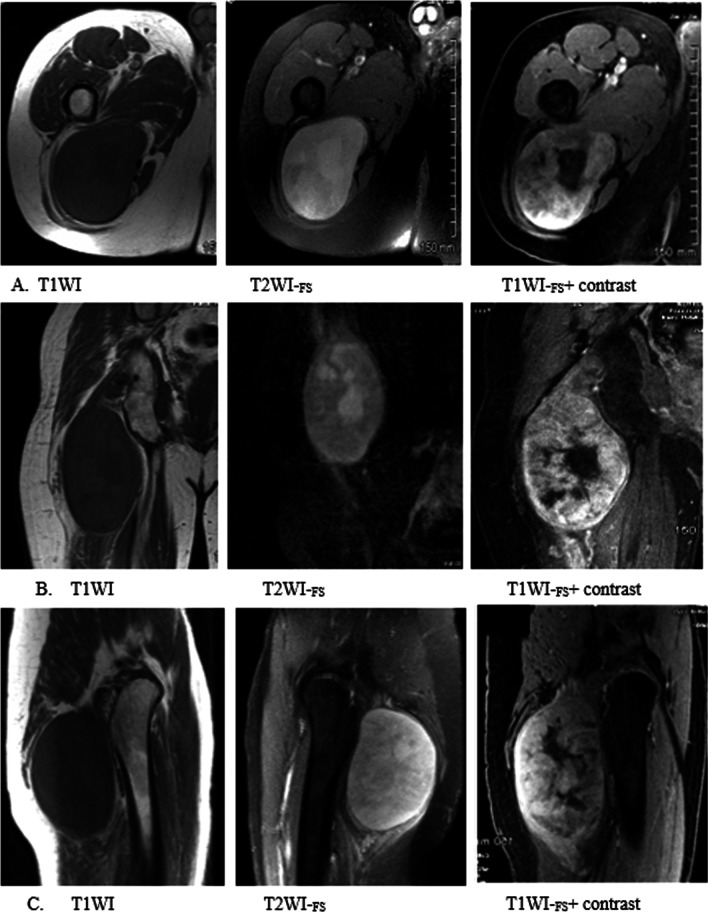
Case 1 MRI of the gluteal and pelvic area. Imaging displays a large heterogeneous enhancing mass in the posterior lateral soft tissues of the right gluteal region. Low-grade peripheral nerve sheath tumor deep to the right gluteus maximus.A- Axial B. Coronal C. Sagittal MRI. T1WI = T1 weighted image. T2WI-_FS_ = T2 weighted, fat-suppressed. T1WI-_FS_ + contrast = T1 weighted, fat suppressed with contrast
